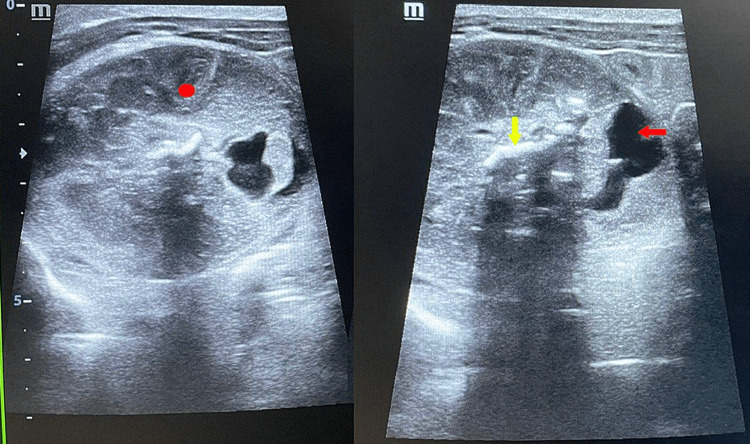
Ultrasound findings taken on postnatal day 2 demonstrated a heterogeneous complex mass with solid echogenic (red solid circle) and cystic anechoic components (red arrow). Osseous elements and linear echogenic areas with posterior acoustic shadowing were also noted within the mass (yellow arrow).
